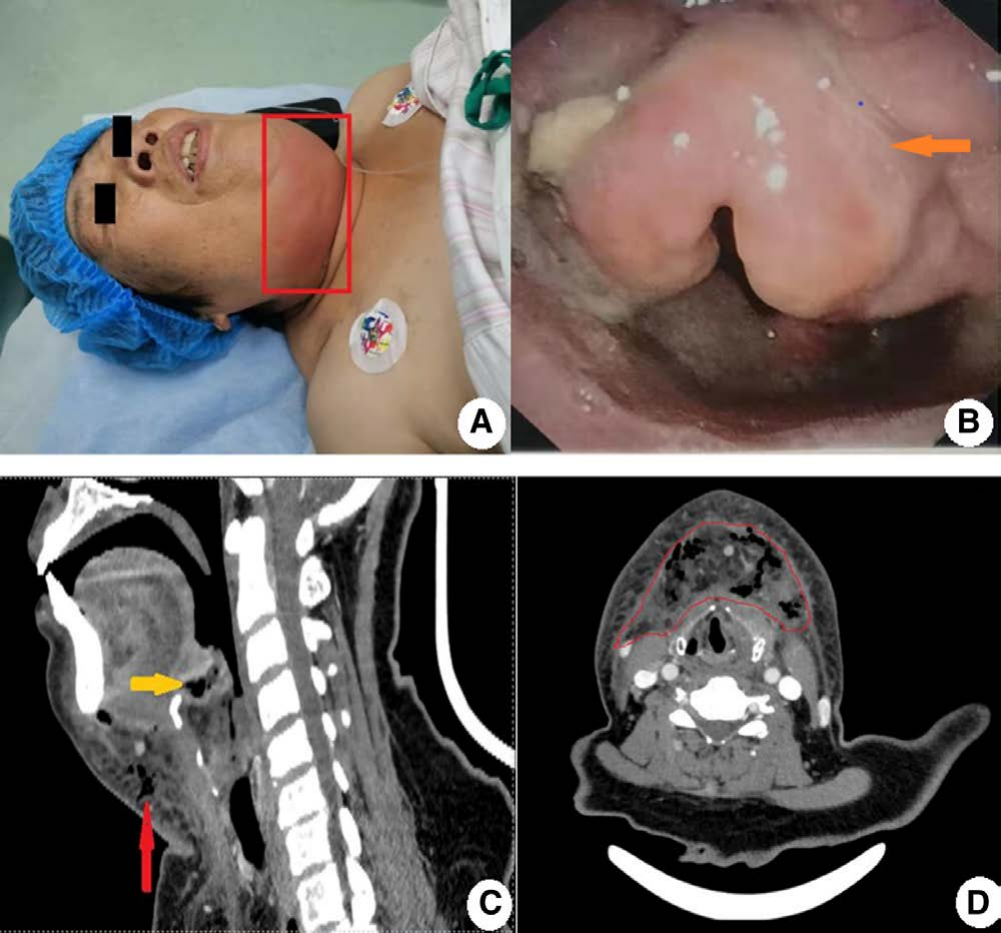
(A) The patient was an old, obese woman with an erythematous and swollen neck. (B) Endoscopic laryngoscopy showed hoof-like, erythematous, and swollen epiglottis with purulent secretions on its surface (orange arrow). (C) Cervical CT scan (sagittal section) showed a swollen epiglottis with abscess cavity (yellow arrow); the air-containing necrotizing tissue in the neck is indicated by the red arrow. (D) Horizontal view of cervical CT scan showing necrotizing tissue in the neck with air and abscess cavity (red area). CT = computed tomography.
